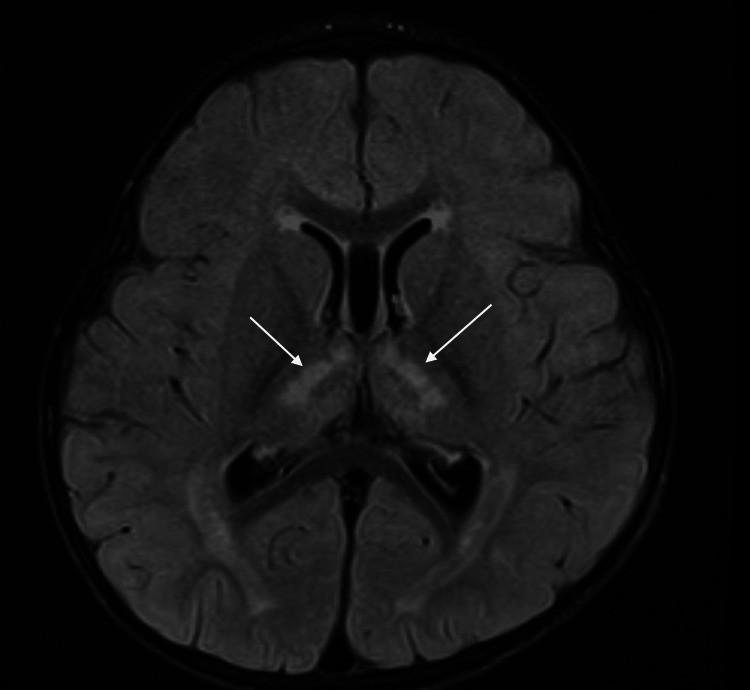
Acute infarction in the left and right thalami with extension inferiorly into the midbrain and pons, T2-FLAIR MRI series. FLAIR: fluid-attenuated inversion recovery
